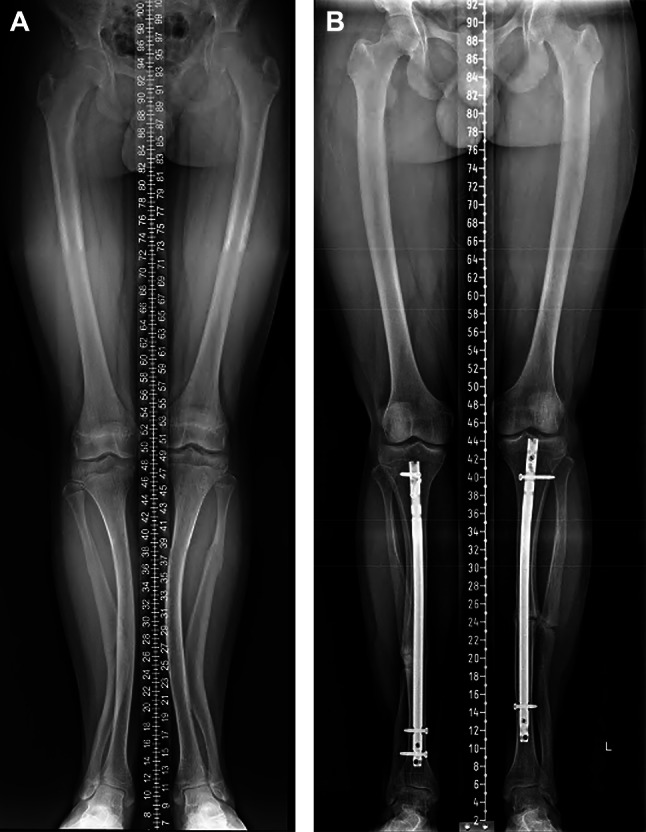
a case of valgus alignment that is tibial in origin; A: pre-op standing full-length radiographs, B: full-lengthen standing radiographs at final fallow-up
